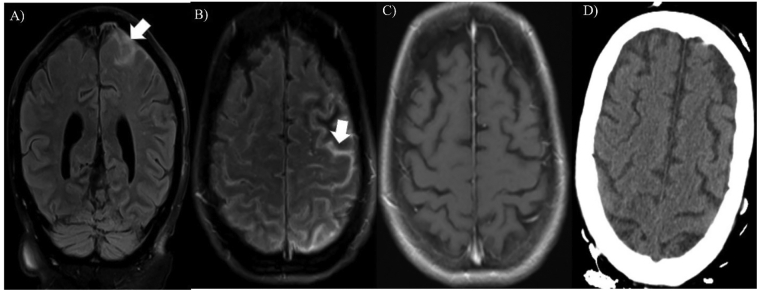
A–B: Coronal and axial FLAIR images, respectively, showing subarachnoid hyperintensity over the left parietal and frontal convexity. C: Axial T1 post-contrast image shows absence of any pathological enhancement. D: Axial brain CT image shows no evidence of subarachnoid blood.
